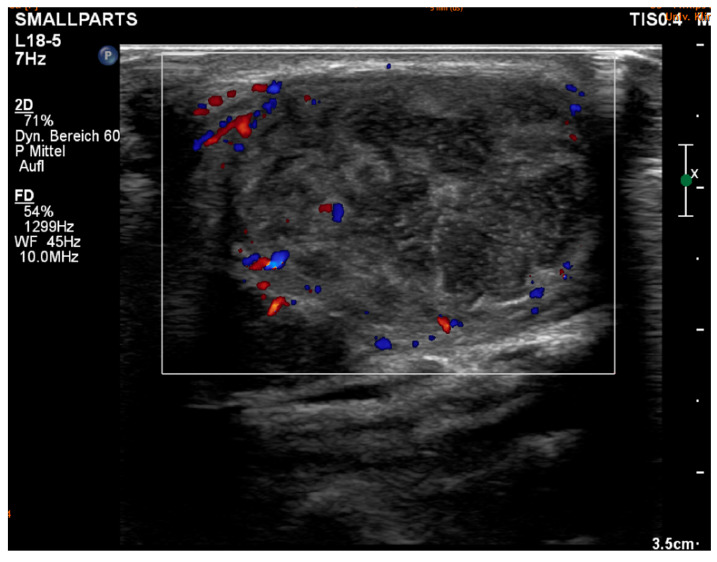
Ultrasound shows enlarged, inhomogeneous lymph nodes. In some cases, the lymph nodes appear liquid; in others, there is extracapsular overgrowth. This appearance suggests an inflammatory altered lymph node in the context of tularemia.
